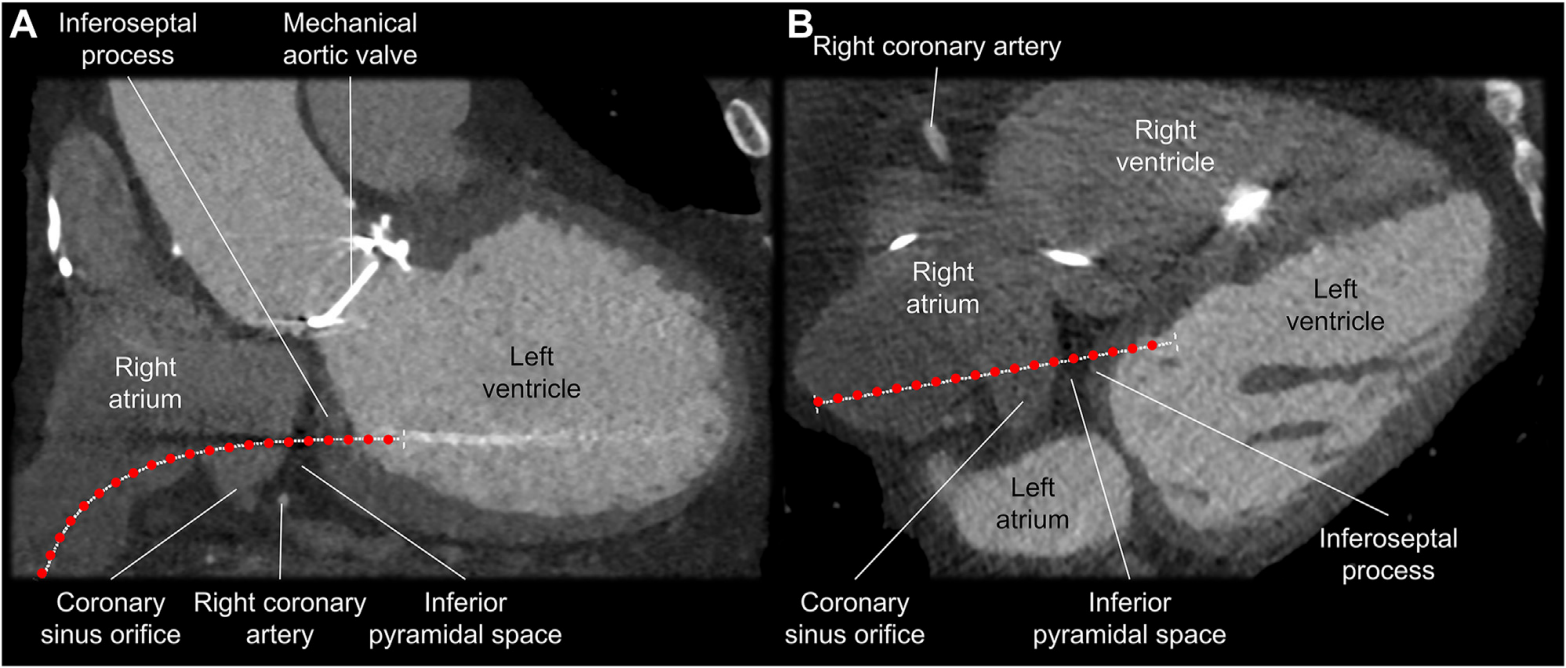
Multiplanar reconstruction images of multidetector-row computed tomography (A: coronal, B: transverse). The expected three-dimensional route (*red dotted line*) of the right atrium to left ventricle puncture was drawn on the multiplanar reconstruction images of computed tomography.
